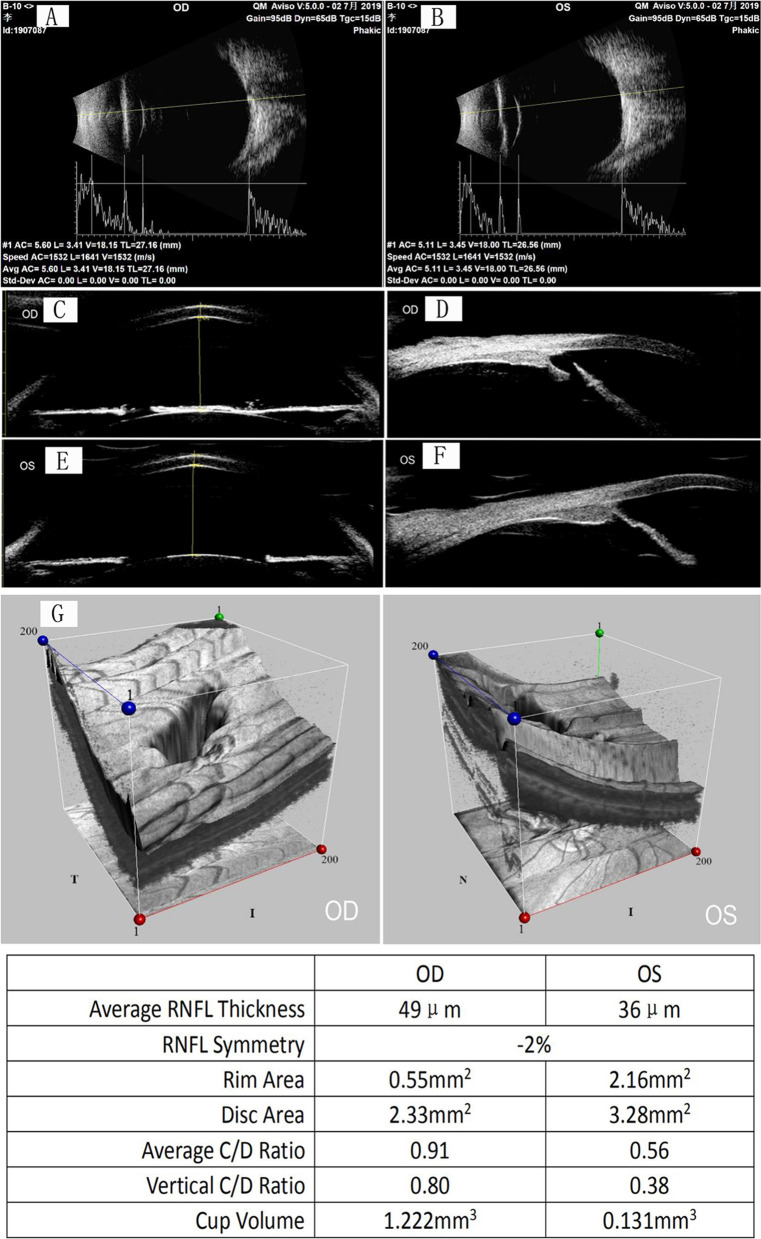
A, B Binocular B-ultrasound images show the prolongation of the axial axis. C–F Binocular UBM images show deepening of the anterior chamber, anterior adhesion, and fracture of the iris. G The OCT images of the binocular disc show that the binocular cup deepened and enlarged due to glaucoma damage
